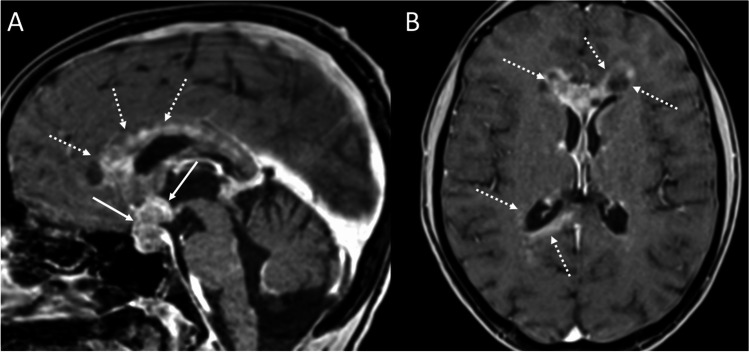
Germinoma with diffuse perivascular spread. Sagittal (A) and axial (B) contrast-enhanced T1-weighted images reveal an intra- and suprasellar mass along with diffuse corpus callosum and periventricular tumoral spread
